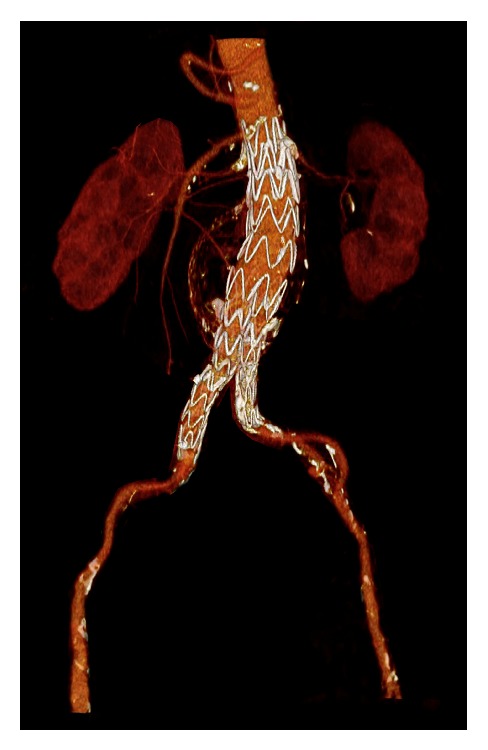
The angio-CT scan at the 3-month follow-up showing patency of renal stents and nice perfusion of both kidneys with no evidence of any endoleak.
